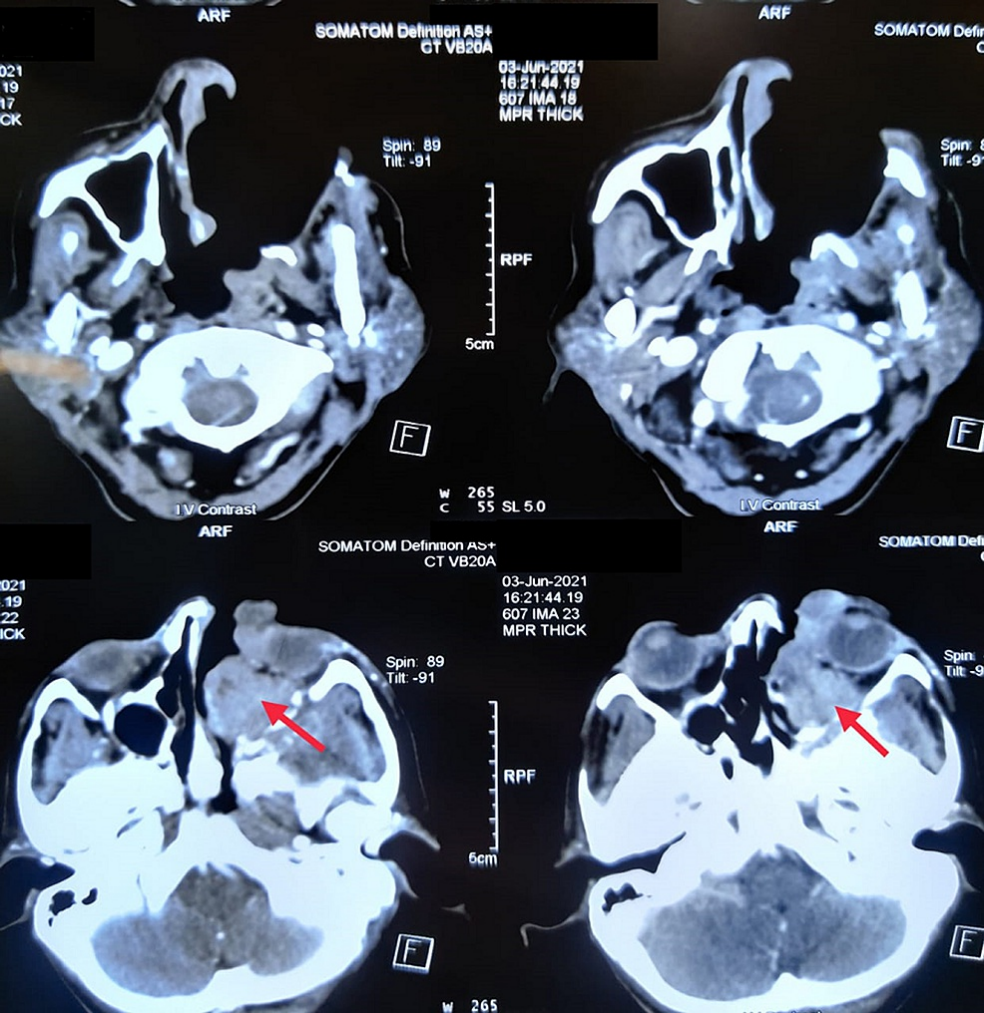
Computed tomography scan of the face showing a left-sided sino-nasal mass surrounding bony destruction and extension to the left orbit
